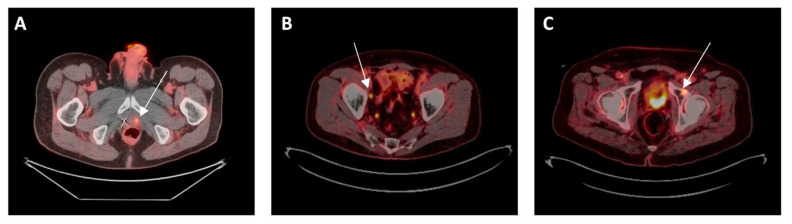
(A) Local failure after prior radiotherapy: 72-year-old man with intermediate-risk prostate adenocarcinoma treated with radiation to the prostate with biochemical failure 5 years later. PSMA PET CT showed uptake in left prostate but no regional or distant disease. He received salvage brachytherapy. (B) Regional failure after prior prostatectomy: 75-year-old man with high-risk prostate cancer treated with radical prostatectomy, with post-op PSA of 0.17, then treated with pelvic and prostate bed radiation. He again had rising PSA and PSMA PET CT showed uptake in one right pelvic lymph node but no local or distant disease. He received stereotactic radiotherapy to the lymph node. (C) Metachronous oligometastasis: 72-year-old man with intermediate-risk prostate adenocarcinoma treated with prostatectomy with undetectable PSA post-op, which then increased a few years later and PSMA PET CT showed uptake in left acetabulum. He received SBRT to the bone lesion without hormone therapy.
